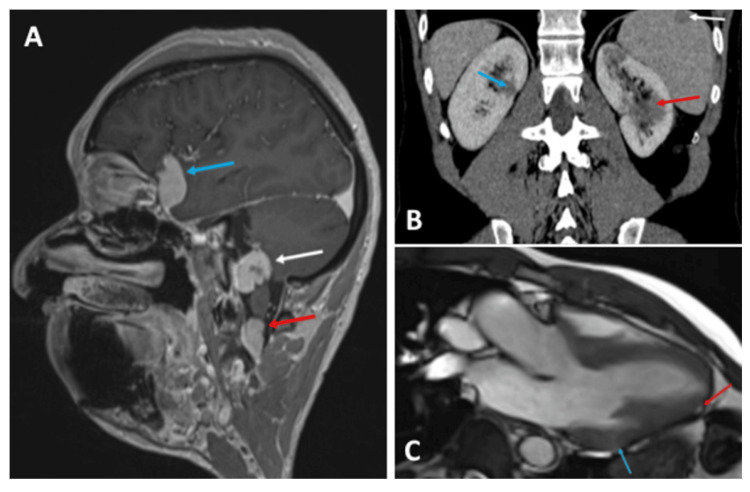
Radiological findings (2019) (A) Brain MRI (enhanced T1sequence) revealing “meningiomatous” cervical (red arrow), infratentorial (white arrow), and supratentorial (blue arrow) lesions. (B) Thoraco-abdomino-pelvic CT scan revealing spleen (white arrow) and kidneys (red and blue arrows) with non-enhanced nodular lesions. (C) Cardiac MRI (T2 sequence) showing endomyocardial infiltrations by focal granulomas next to the apex (red arrow) and the anterolateral papillary muscle (blue arrow).
